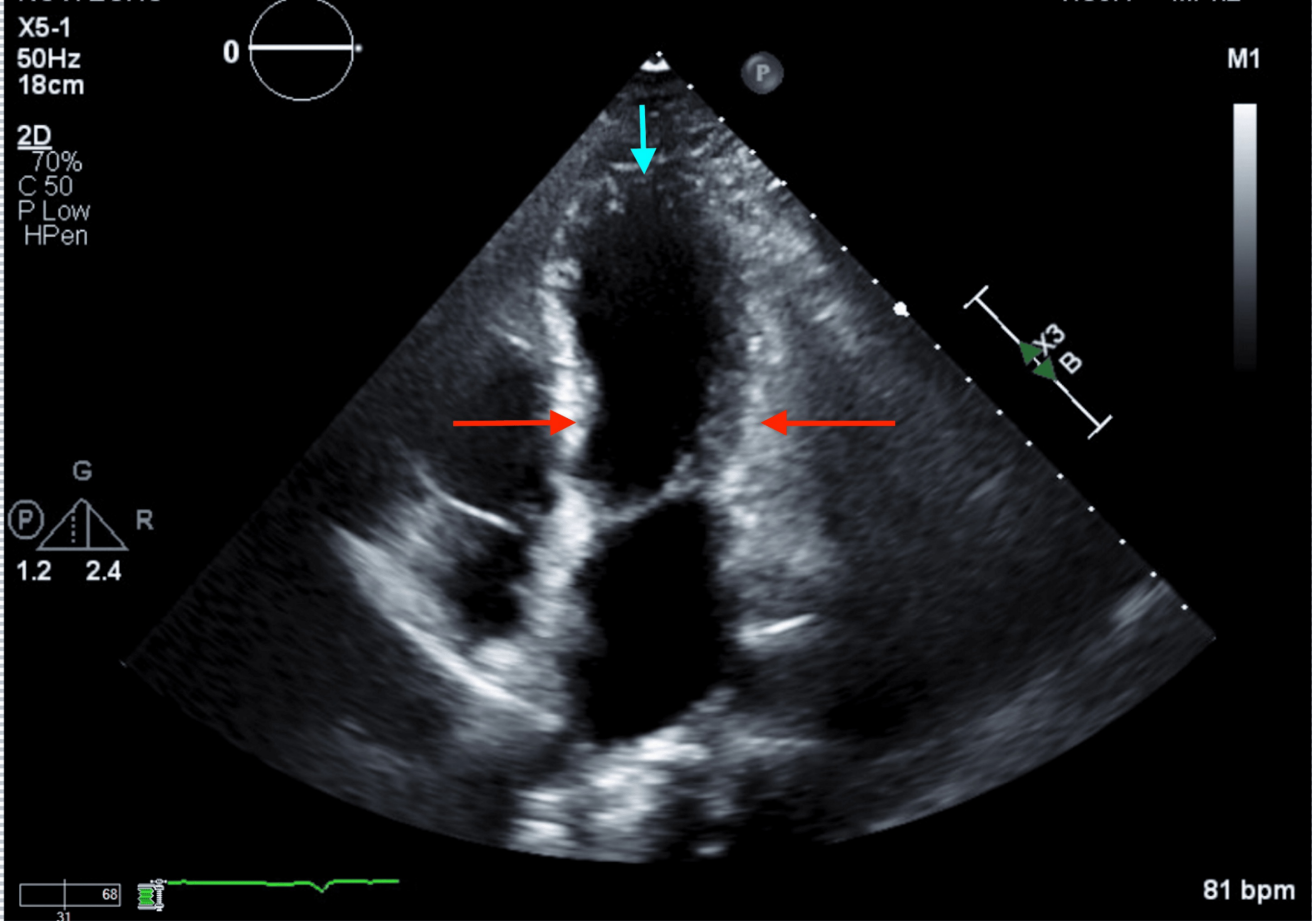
Apical four-chamber view on transthoracic echocardiography with hyperkinesis of the basal segments (red arrows) and apical ballooning (light blue arrow) concerning Takotsubo cardiomyopathy.
